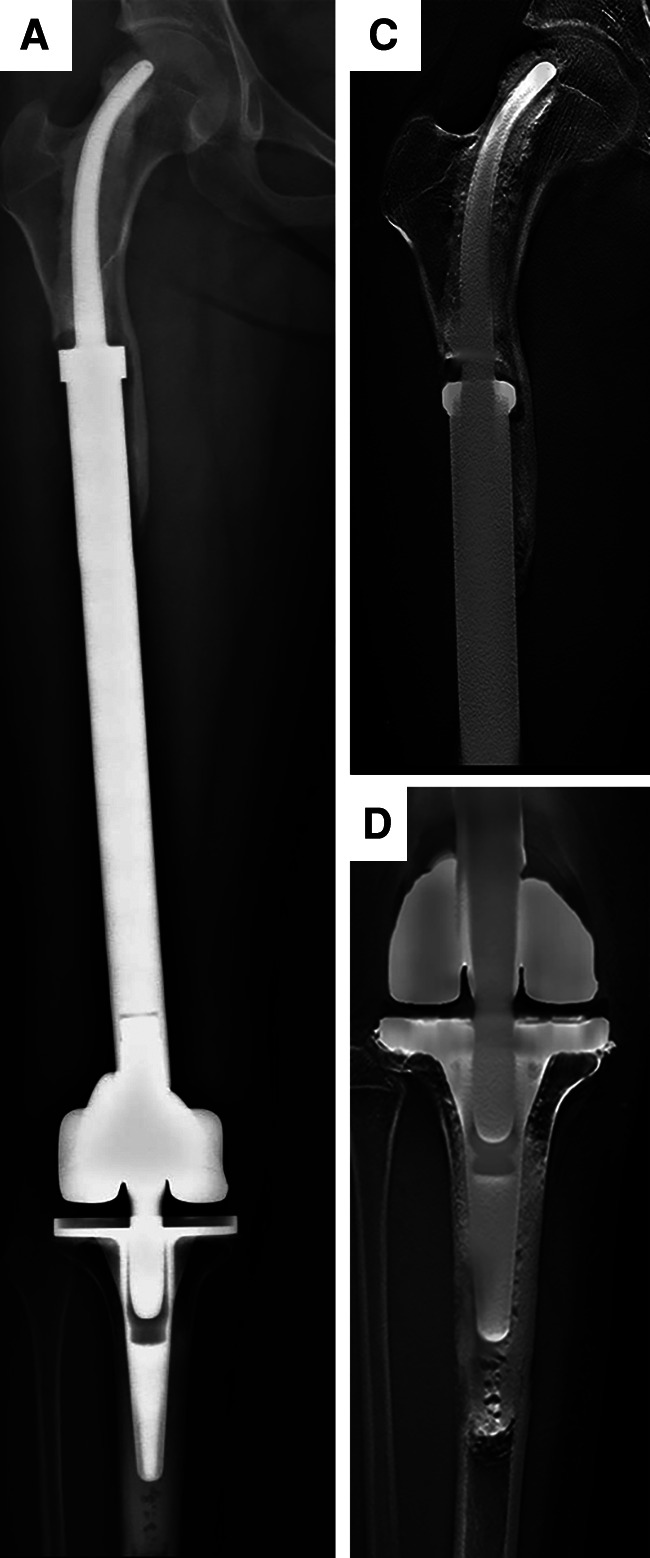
Radiographs showing the 102-month postoperative views of the customized cemented femoral endoprosthesis with an intra-neck curved stem placed during treatment for an osteosarcoma. (A) Posteroanterior radiograph of the entire femur. (B) Posteroanterior with Shimadzu Metal Artefact Reduction Technology (*T*-smart) views of the stem insertion region in the proximal femur. (C) Posteroanterior *T*-smart views of the stem insertion region in the proximal tibial.
